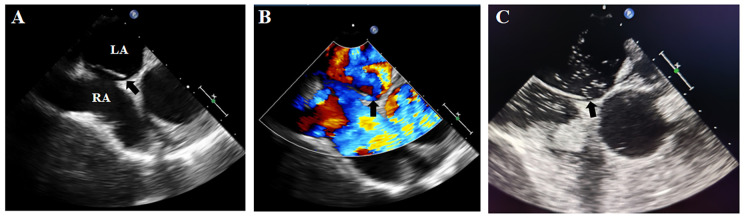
Transesophageal echocardiographic findings. (A,B) Demonstrated a significant PFO with right-to-left shunting; (C): strongly positive bubble study. Black arrow: PFO. LA, left atrium; RA, right atrium.
